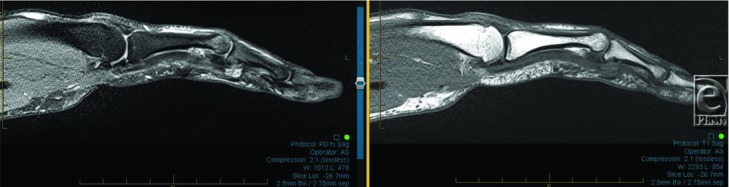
Lateral magnetic resonance scans at the second recurrence following excision showing the lesion on T1- and T2-weighted images.
